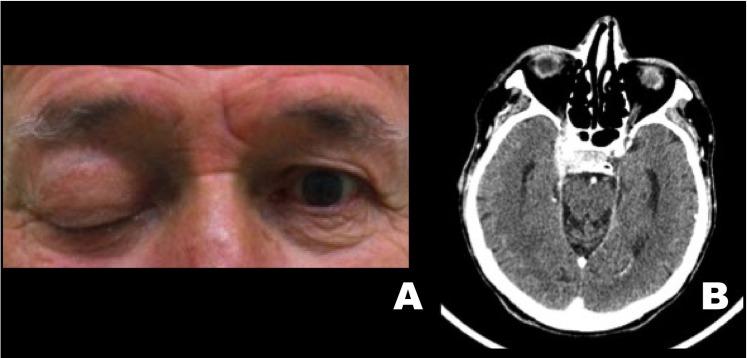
A. Extraoral clinical aspect showing right eyelid ptosis. B. Axial CT (soft-tissue window display) demonstrating an extensive hyperdense image at the base of the skull associated with the sphenoid bone.
